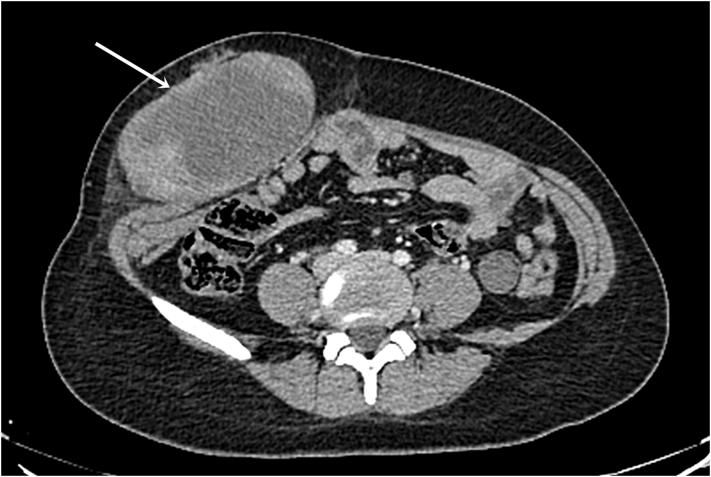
CT scan in the axial plane showing a well-circumcised, heterogeneous soft tissue mass of the abdominal wall (arrow).
